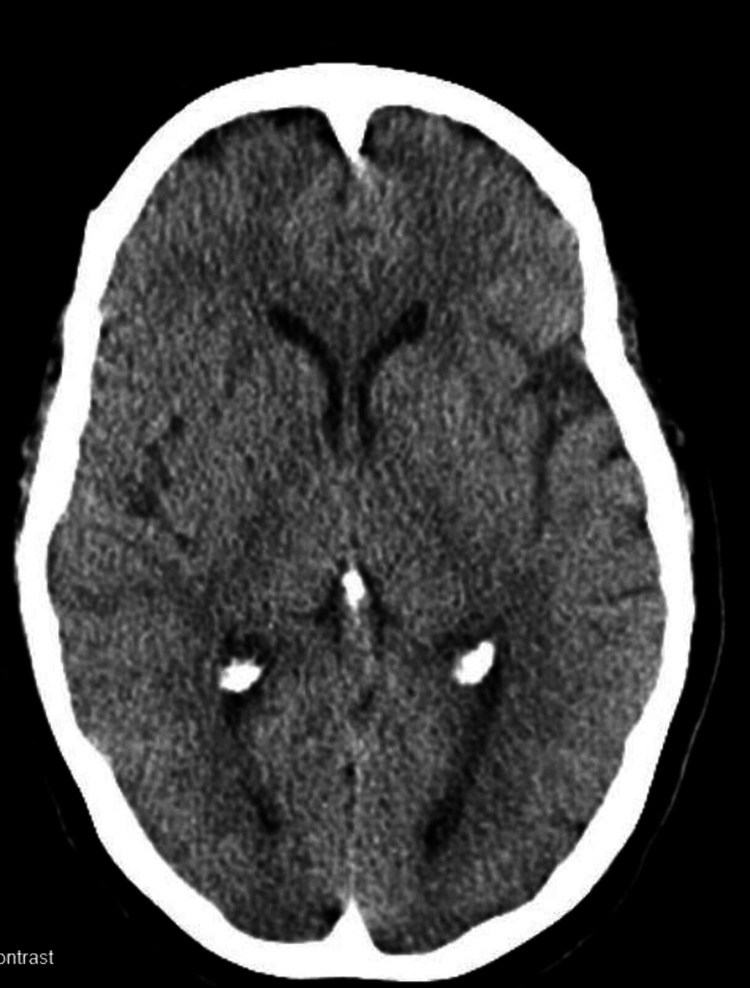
A CT of the head without contrast revealed no acute intracranial abnormalities.
